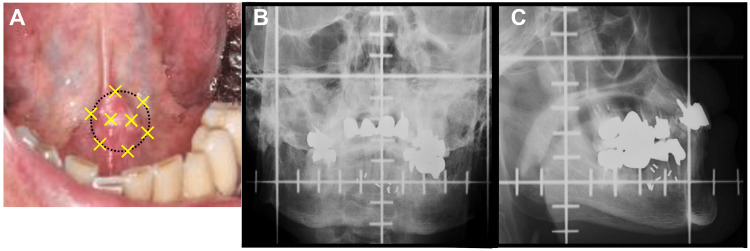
Implant information. Treatment target area (black dotted circle) and distribution of Au-198 grains (yellow cross) (A). Actual distribution of Au-198 grains in posteroanterior (B) and lateral (C) X-ray photographs.
